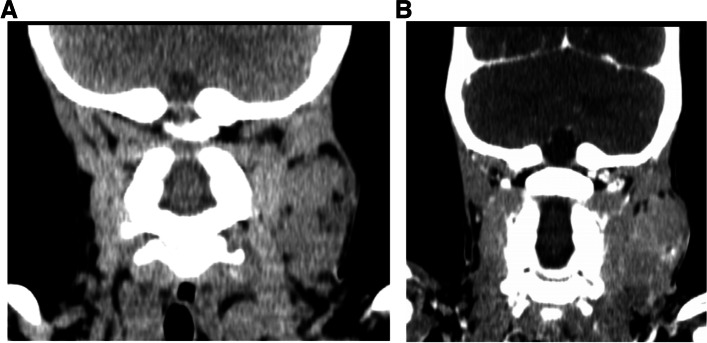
A An unenhanced coronal CT of the head and neck. There was multiple enlarged left level II, III, IV and V lymph nodes of up to 3.5 cm. B A contrast enhanced coronal CT, which demonstrated enlarged poorly enhancing left level II, III, IV and V lymph nodes. A diagnosis of lymphoma was made on both A and B
